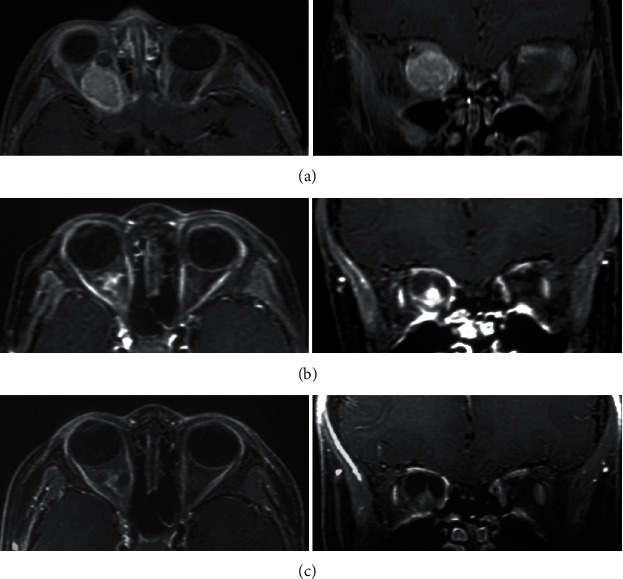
Contrast-enhanced MRI of pediatric OPG before and after single fraction GKS (16.0 Gy). A male OPG patients aged 30 months was observed with proptosis of the right eye, accompanied with VA damage for more than 2 months. (a) MRI at GKS. The tumor is located in the right orbit and surrounded the optic nerve and was homogeneous enhanced on MRI. The baseline VA was 0.15 on the right. (b) Three years after GKS, the tumor volume was smaller than baseline, there was intratumoral necrosis and cyst formation, and tumor enhancement effect declined. The VA remained 0.15, and the proptosis improved significantly. (c) Five years after GKS, the tumor volume remained stable, and tumor enhancement effect declined continuously. The VA was less than 0.1.
